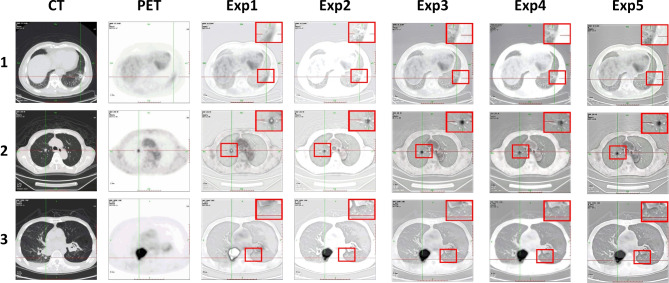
The ablation experiments fusion results of CT lung window images and PET images.
